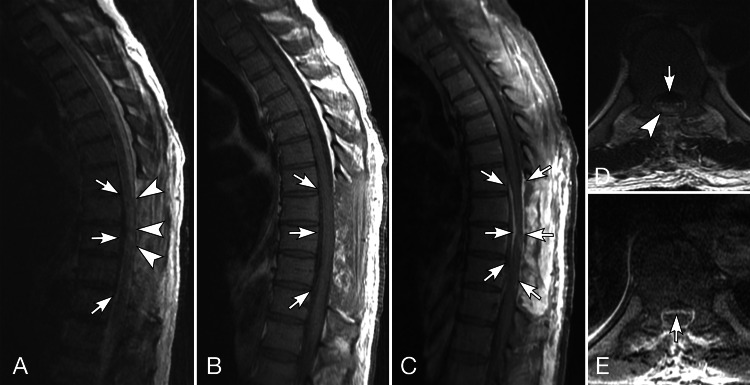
MRI of the thoracic spine after decompression. Sagittal T2 (A) and T1 pre-contrast (B) and T1-weighted fat-suppressed post-contrast (C) MRI, and axial T2 (D) and fat-suppressed post-contrast T1-weighted (E) MRI. The axial images are at T8-T9. Posterior decompression has been performed at T7-T10. A relatively thin T2 and T1-hypointense lesion is present in the ventral aspect of the spinal canal T7-T11 (white arrows in A, B, D). This has decreased in size since the comparison CT. As best seen on the axial images, this has a dural rather than epidural appearance. Circumferential dural enhancement (white arrows in C and E) is greatest ventrally, along the lesion’s dorsal surface. The spinal cord is compressed ventrally and contains abnormal T2 hyperintensity spanning across the T8 and T9 vertebral body levels (black arrows in A, D).
